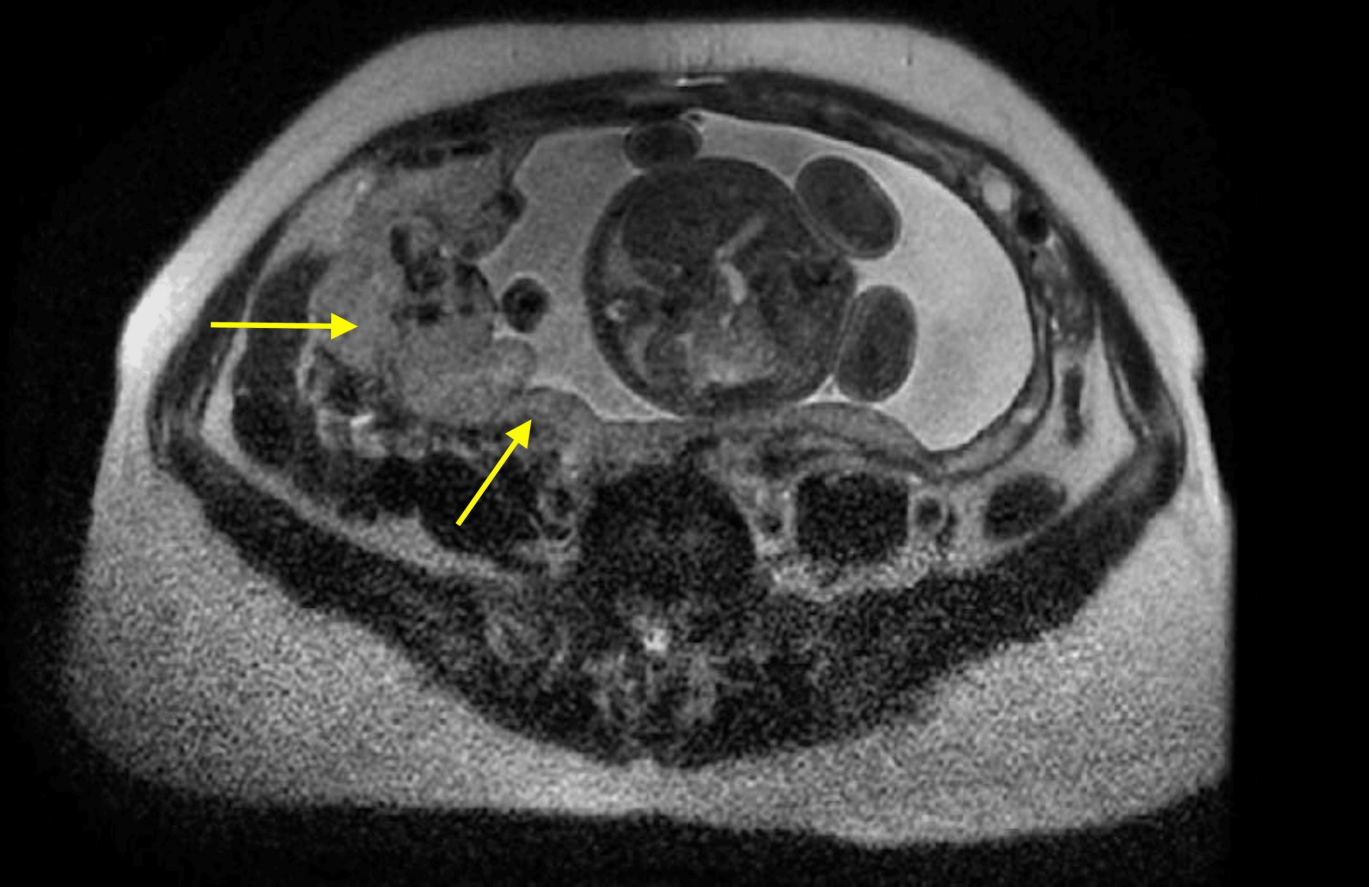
Axial T2-weighted image with arrows pointing at heterogeneously hyperintense fundo-posterior myometrium of the uterus.
